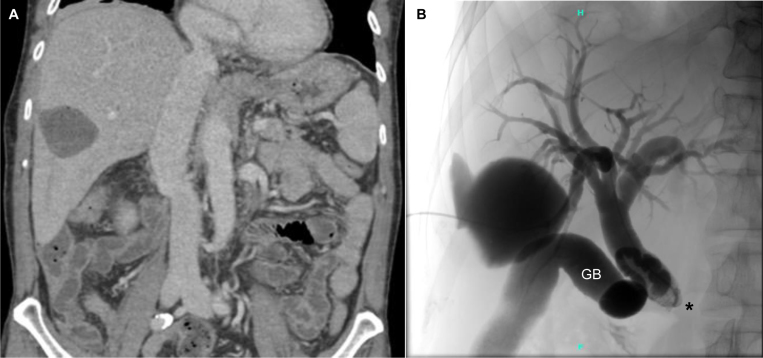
CT image shows a post-RFA biloma formation (A), during percutaneous biliary access the surgical anastomosis (*asterisk*) could not be traversed, an external PTD was placed temporarily (B). CT, computed tomography; GB, gallbladder; RFA, radiofrequency ablation; PTD, percutaneous transhepatic biliary drainage.
